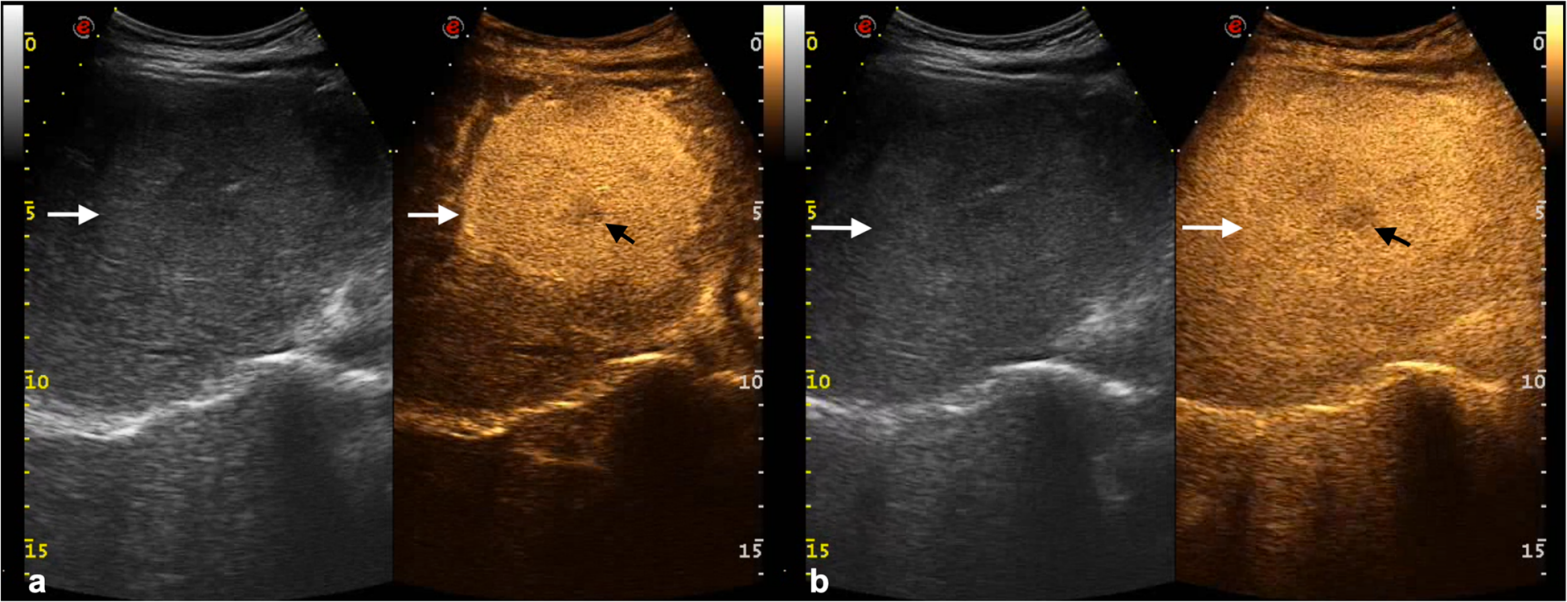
Contrast-enhanced ultrasound for assessment of focal nodular hyperplasia. Dual-display images with brightness-mode (left) and contrast-enhanced ultrasound images (right). a Images in arterial phase (28 s after the i.v. injection of contrast agent) show homogeneous and strong enhancement (white arrows) with a central hypoechoic scar (black arrow). b In the portal venous phase (86 s after the injection), the lesion is still slightly hyperechoic to the adjacent liver parenchyma (white arrows) and the central scar remains hypoechoic (black arrow)
